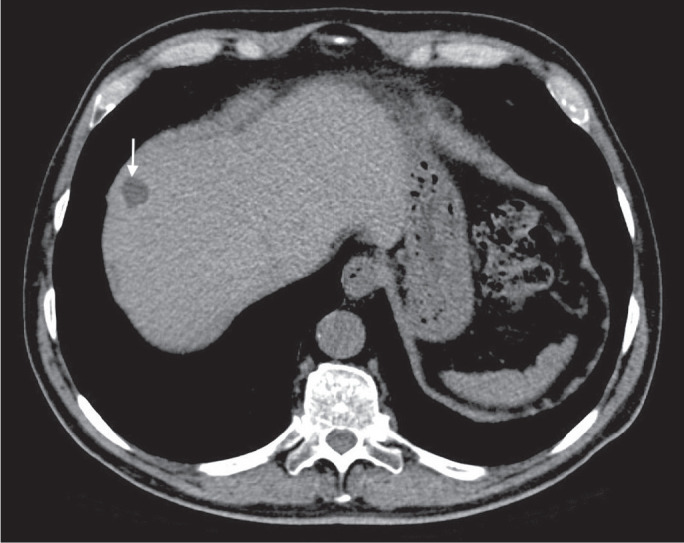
Chest CT of a 44-year-old male, showing a small, well-defined lesion
